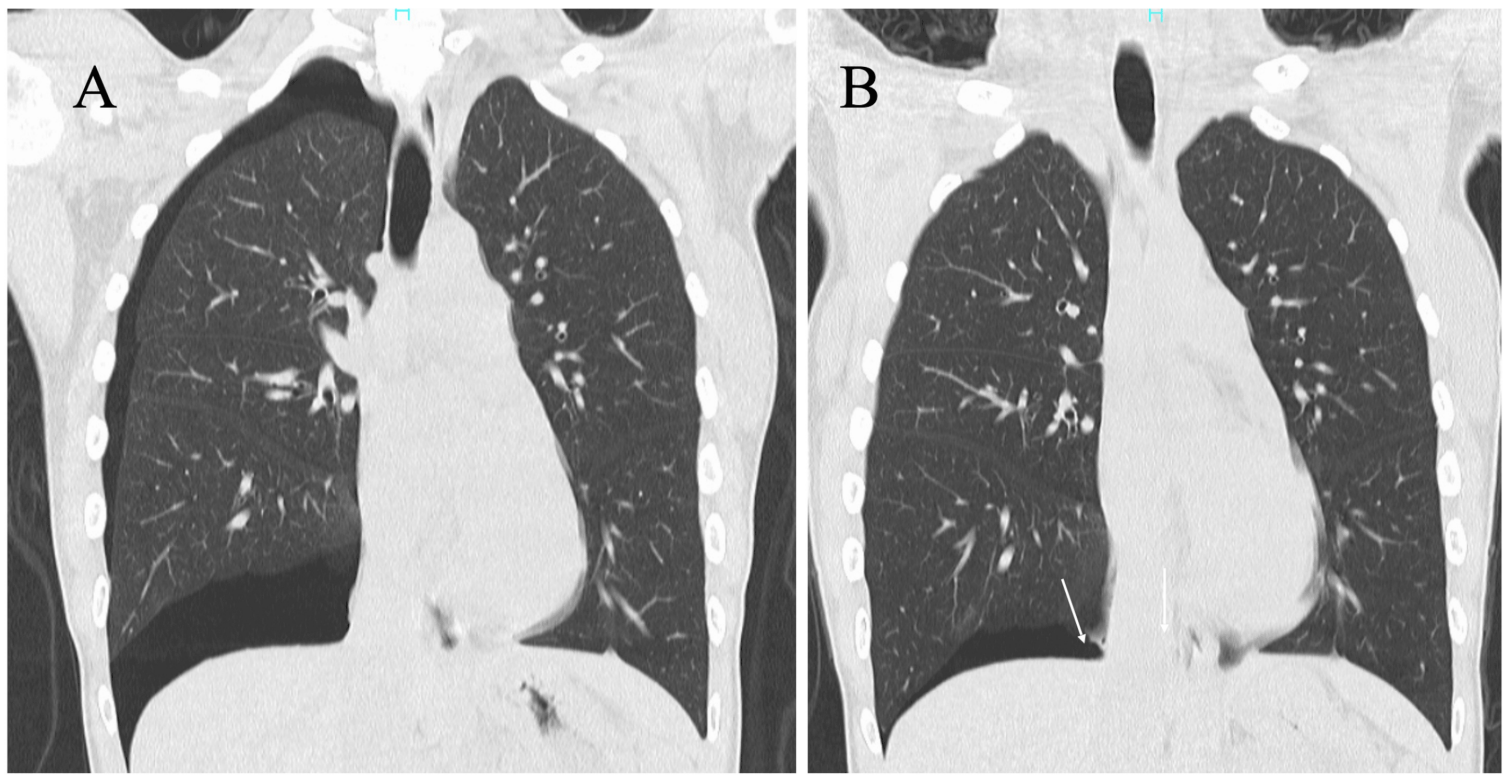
(A) Coronal computed tomography (CT) showed mild circumferential collapse of the right lung at her first visit to our hospital. (B) Coronal CT showed a small air space just above the right diaphragm at the pneumothorax recurrence.
